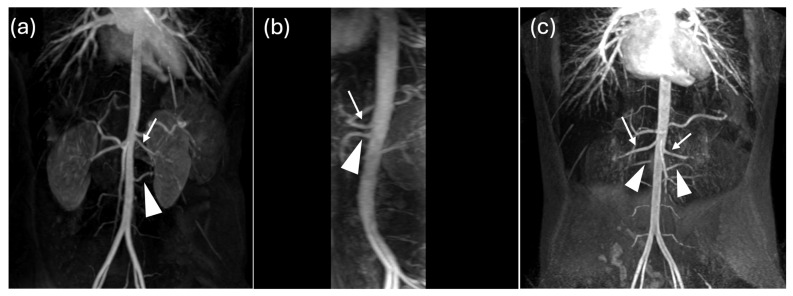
MIPr of Contrast Enhancement Magnetic Resonance Angiography. MR images show the main renal arteries (arrows) and an accessory artery (arrowhead) directed to the left (a), right (b) and both (c) kidneys.
